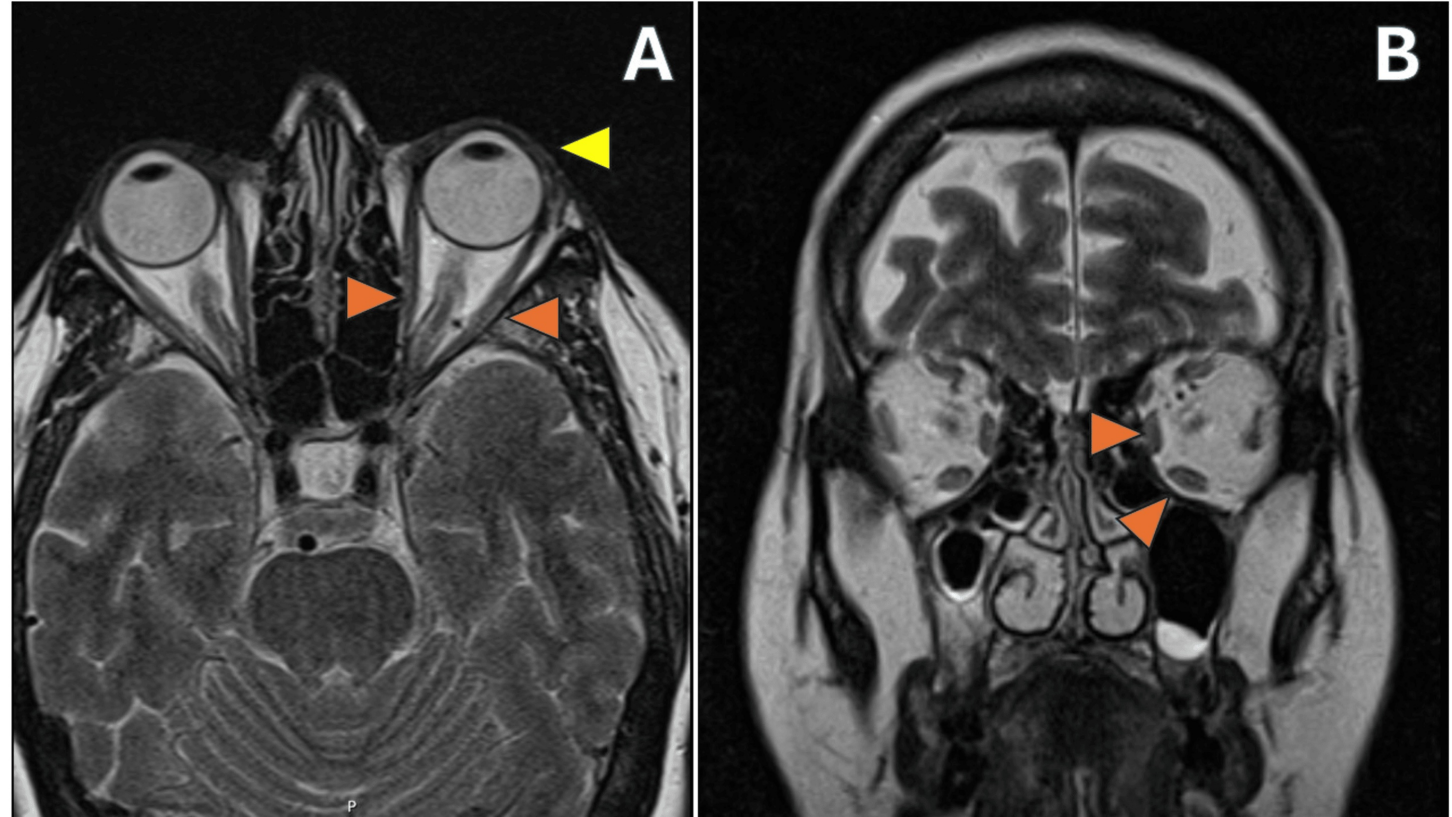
Orbital MRIs Panel A (axial T2-weighted sequence): Mild inflammation of the extraocular muscles can be observed, characterized by increased signal intensity in the muscle mass, sparing the tendon, highlighted by orange arrows. Anterior protrusion of the left globe is also noted, indicated by the yellow arrow. Panel B (coronal T2-weighted sequence): Hypertrophy of the left extraocular muscles, as indicated by the orange arrows. MRI, magnetic resonance imaging
